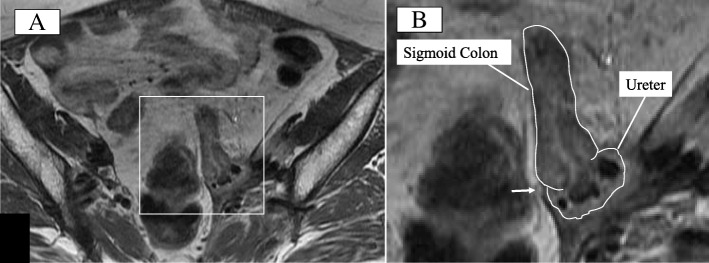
Magnetic resonance imaging revealed a fistula formation (arrow) between the sigmoid colon and ureter (a overall view and b enlarged view)
